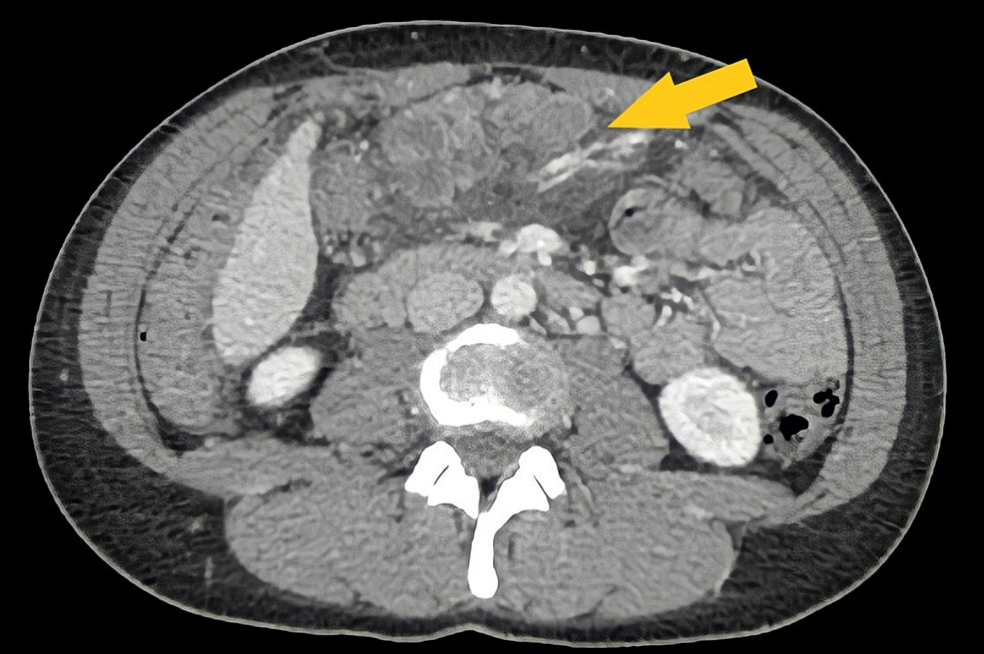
CT abdomen: axial plane, portal venous phase. The arrow indicates epigastric lesion CT: computed tomography
